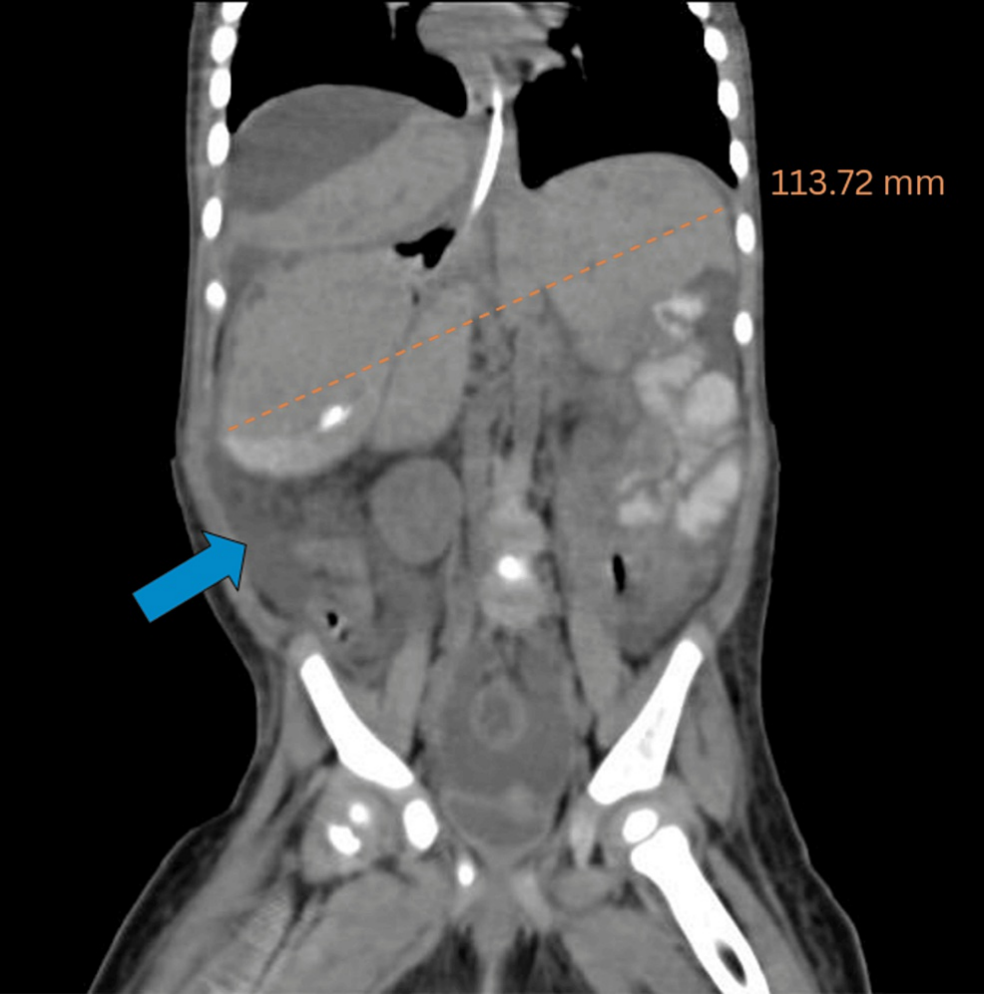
Coronal view of abdominal CECT showing hepatomegaly (orange dotted line) and ascites (blue arrow). CECT: contrast-enhanced computed tomography
